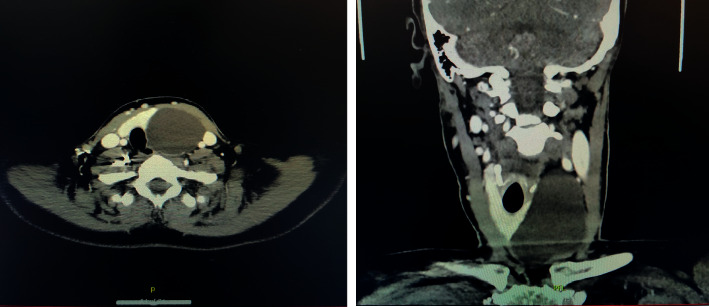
CT neck demonstrating left thyroid cystic mass measuring 7.9 × 5.7 × 5.7 cm displacing left internal jugular vein and left common carotid laterally.
